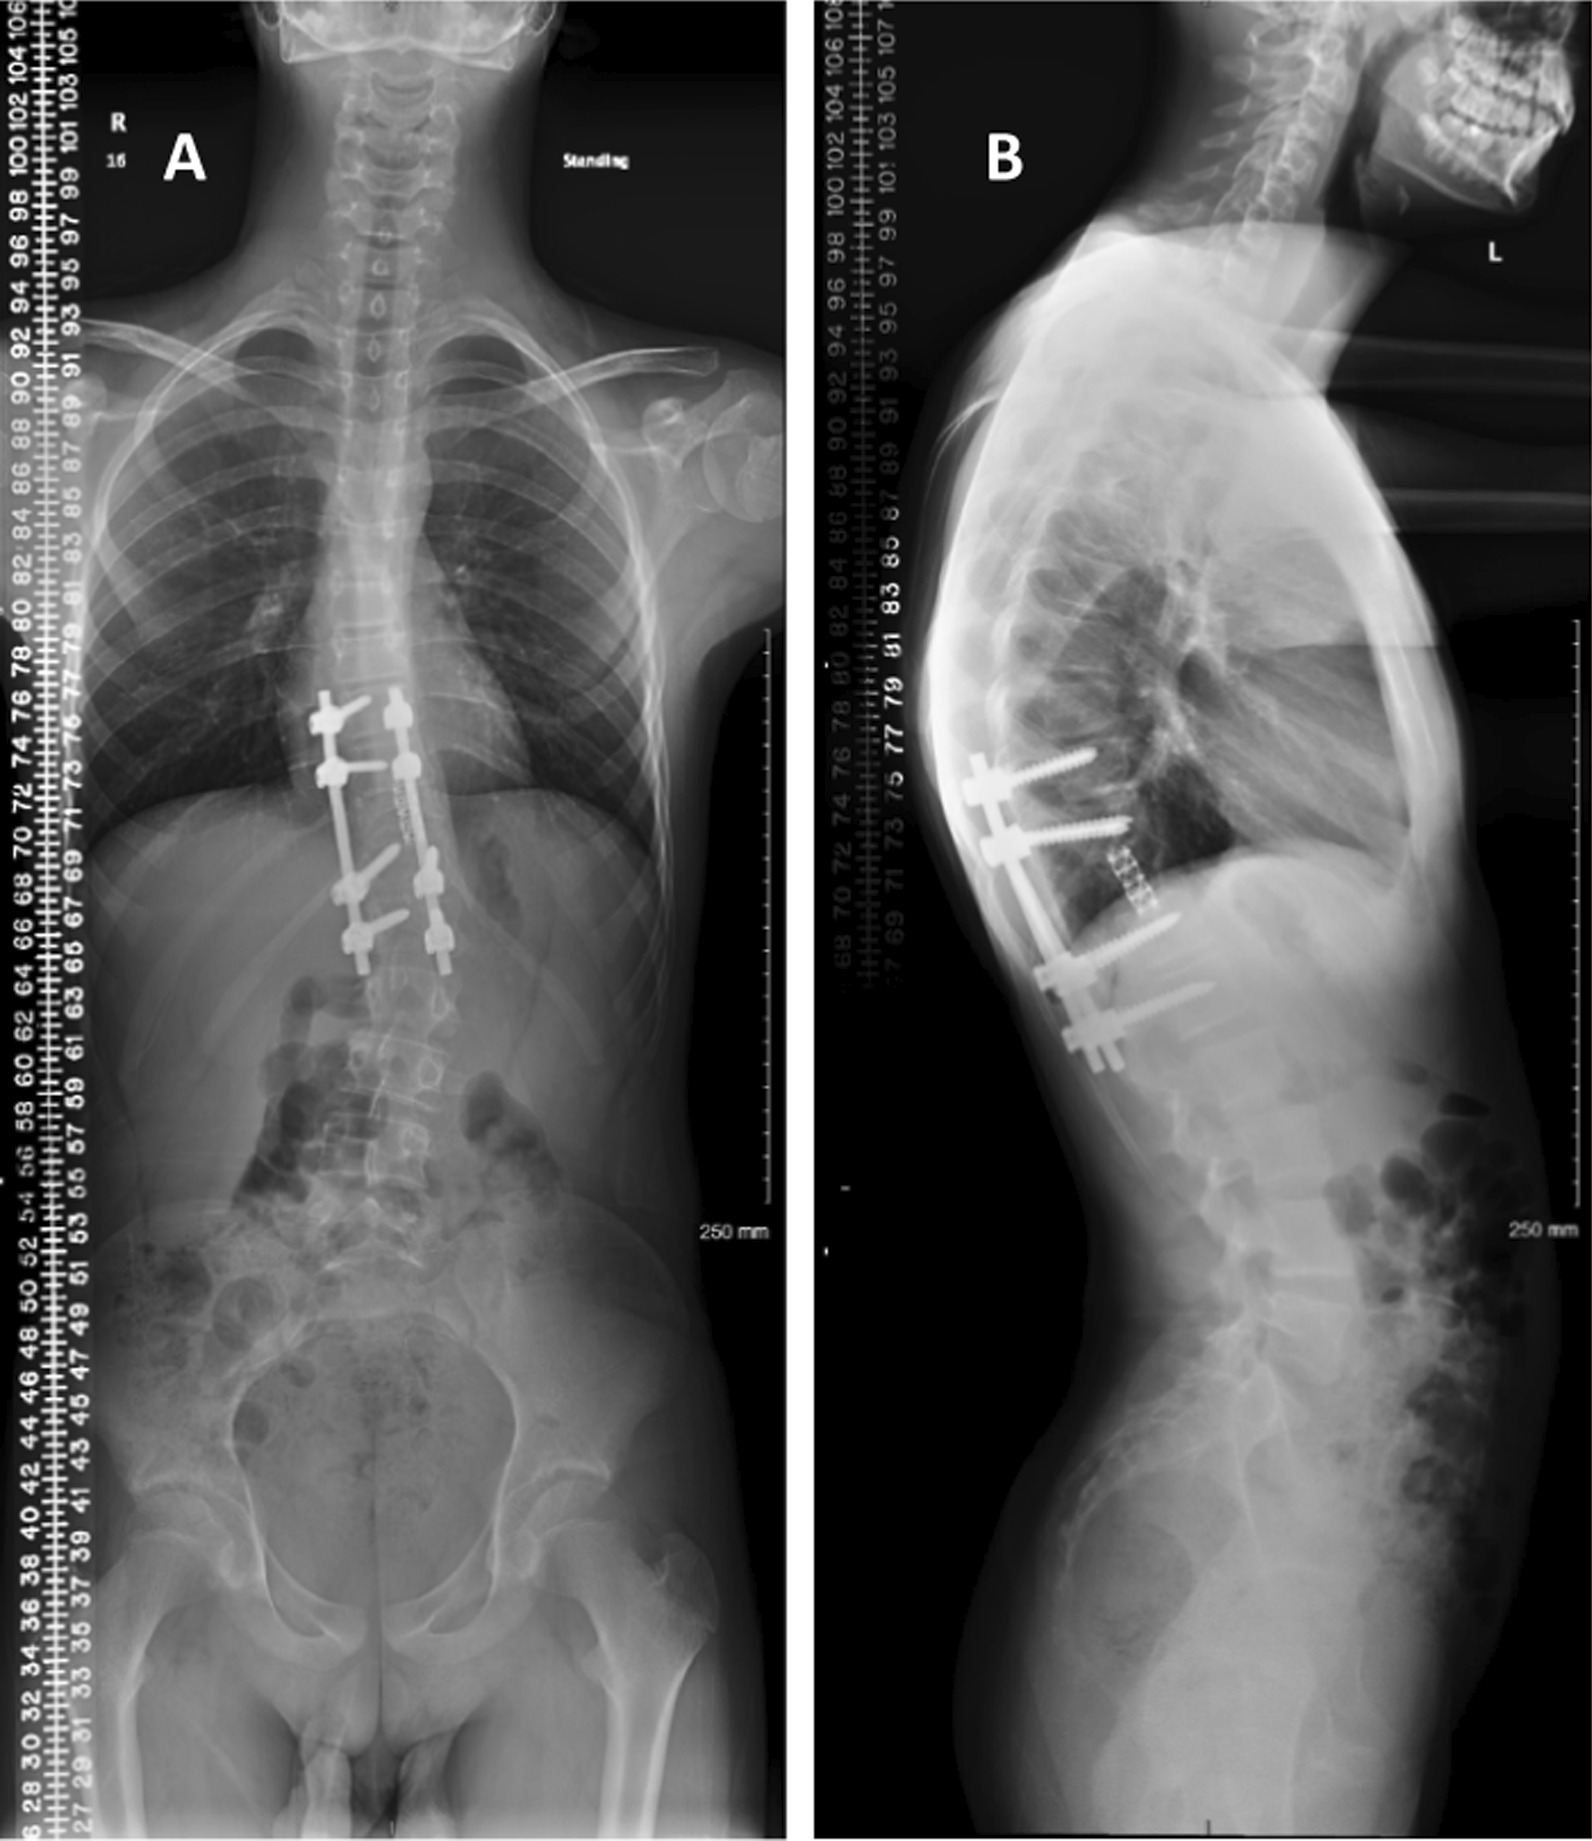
A, B Scoliosis X-ray A AP and B lateral, 2 years post first surgery showing T11 resected vertebral body with cage placement thoracolumbar fixation rods and screws with S-shape scoliosis with Cobb angle of 30°
